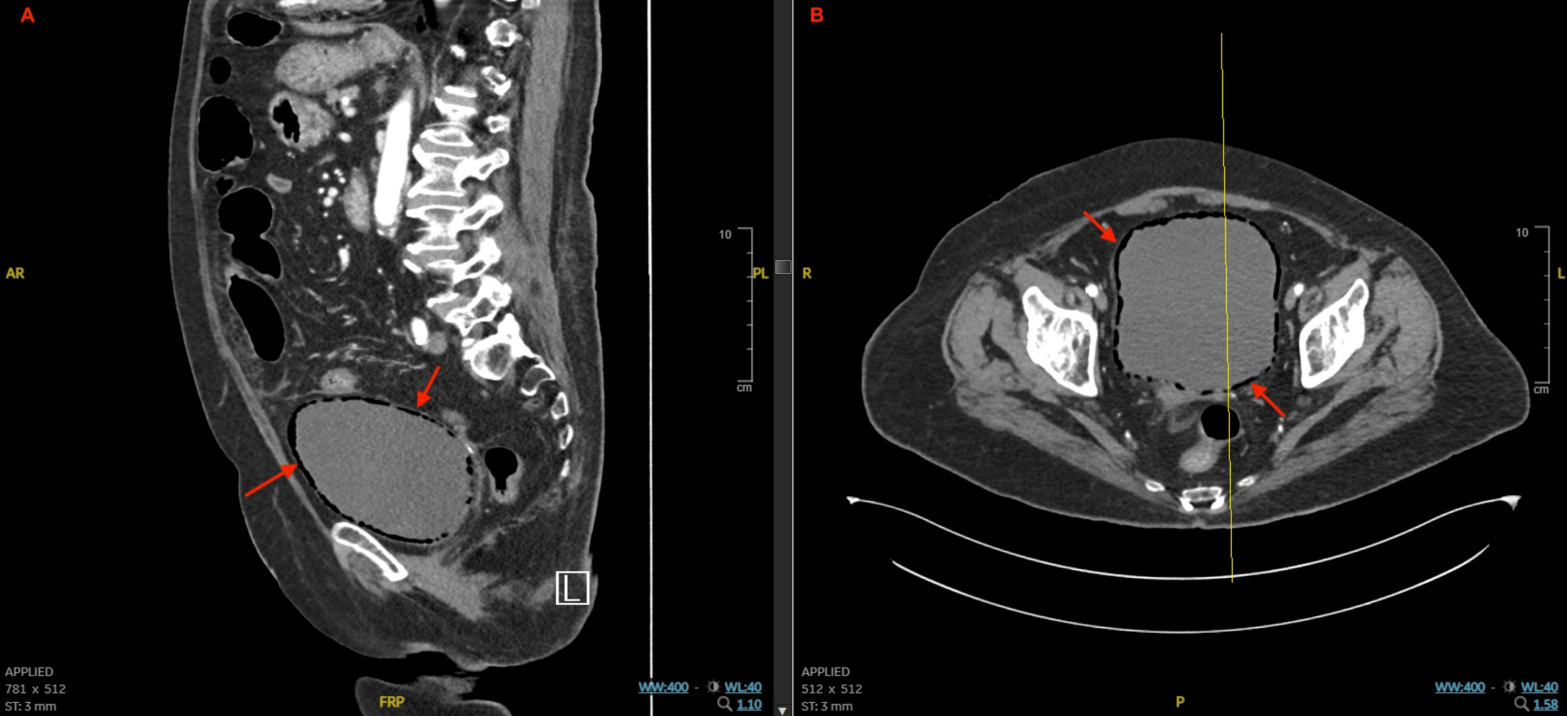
Sagittal and axial CT views of emphysematous cystitis. (A) Sagittal CT angiography showing gas tracking circumferentially within the bladder wall. (B) Axial CT angiography confirming intramural gas throughout the bladder wall. No extraluminal extension or associated abscess is observed.
